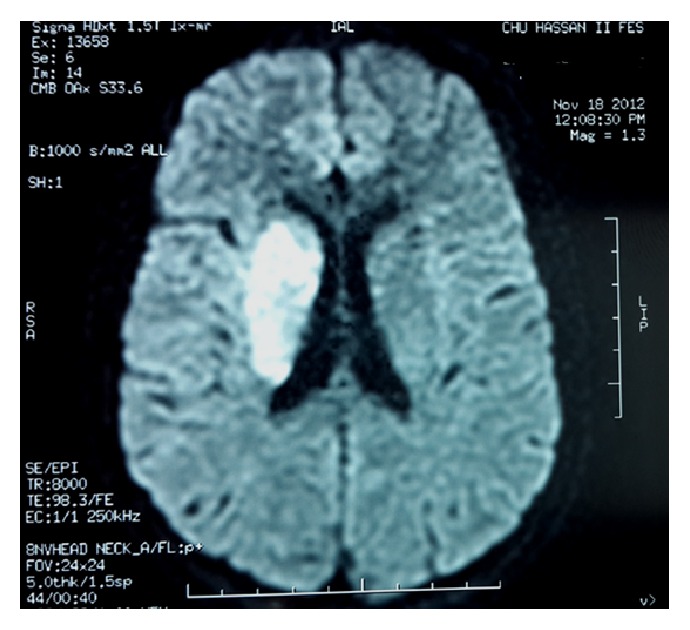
MRI Echo-Planar Imaging (EPI) sequence showing a right middle cerebral infarction.
